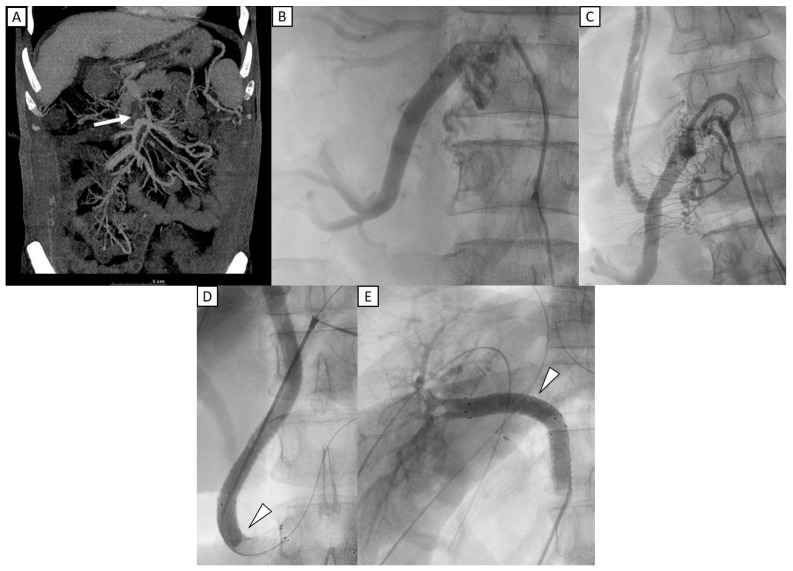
A 48-year-old man who underwent liver transplantation. (A) Preoperative CT imaging revealed total occlusion of portal vein with thrombosis of the superior mesenteric vein (SMV) (white arrow). (B) IOPV through the mesenteric vein confirmed complete occlusion of the distal SMV, with no flow to portal vein, and with a large ileocolic vein. (C) An interposition graft with Gelsoft (10 mm, 25 cm) (Terumo, Tokyo, Japan) was placed from the ileocolic vein to the grafted right portal vein. Multiple filling defects were identified within the interposition graft, favoring thrombi. (D,E) Two stents (12 mm × 60 mm) (E-Luminexx, Bard Peripheral Vascular, Tempe, AZ, USA) were deployed, with one extending from ileocolic vein to the interposition graft and another from the interposition graft to the grafted right portal vein (arrowhead). Post stenting portal venography confirmed patency of the interposition graft and portal vein.
